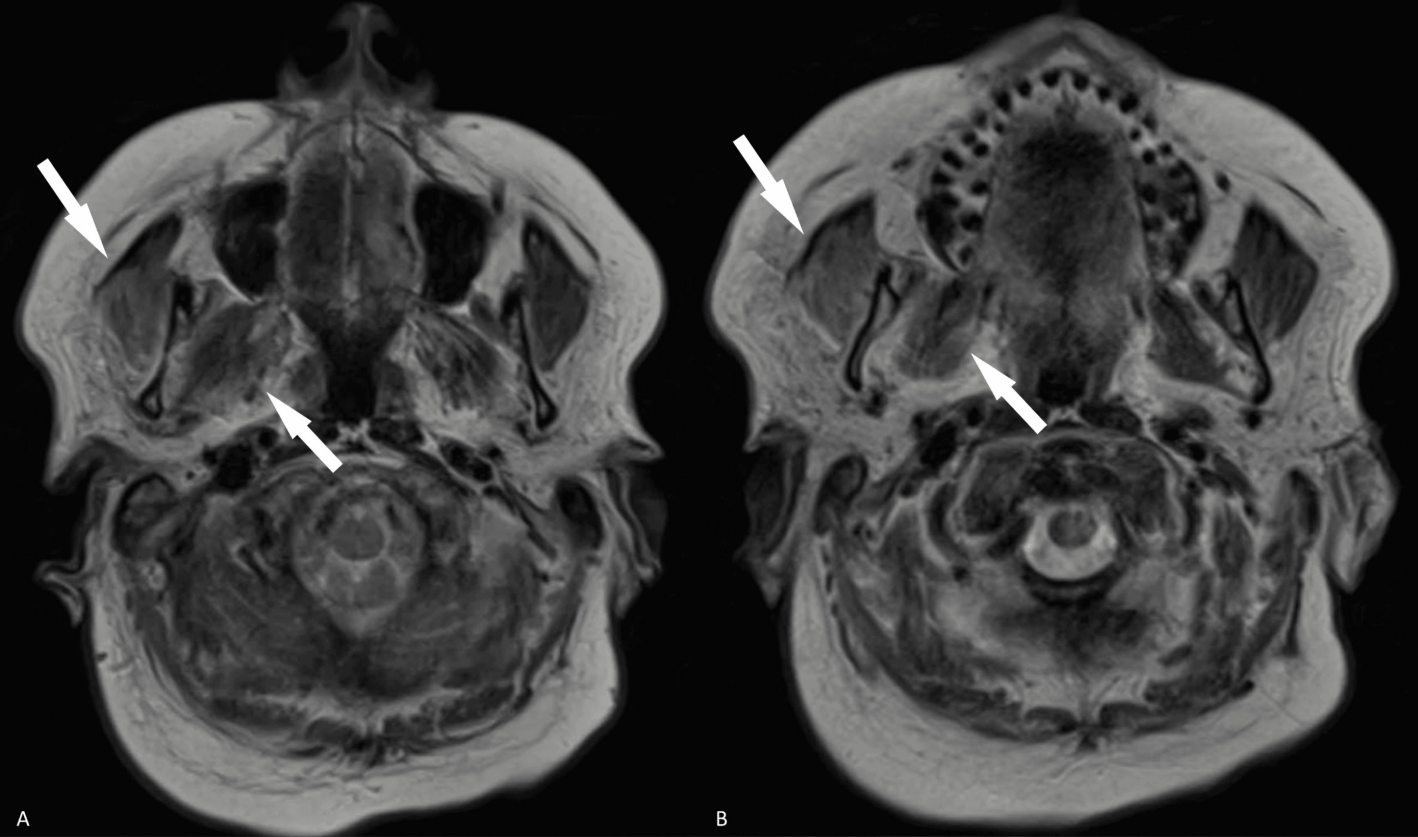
MRI images of the brain showing findings of resolved right masticator space cellulitis. Axial T2-weighted images demonstrate resolution of abnormal T2 signal intensity within the muscles of the right masticator space (white arrows, A and B) compatible with resolved right masticator space cellulitis.
